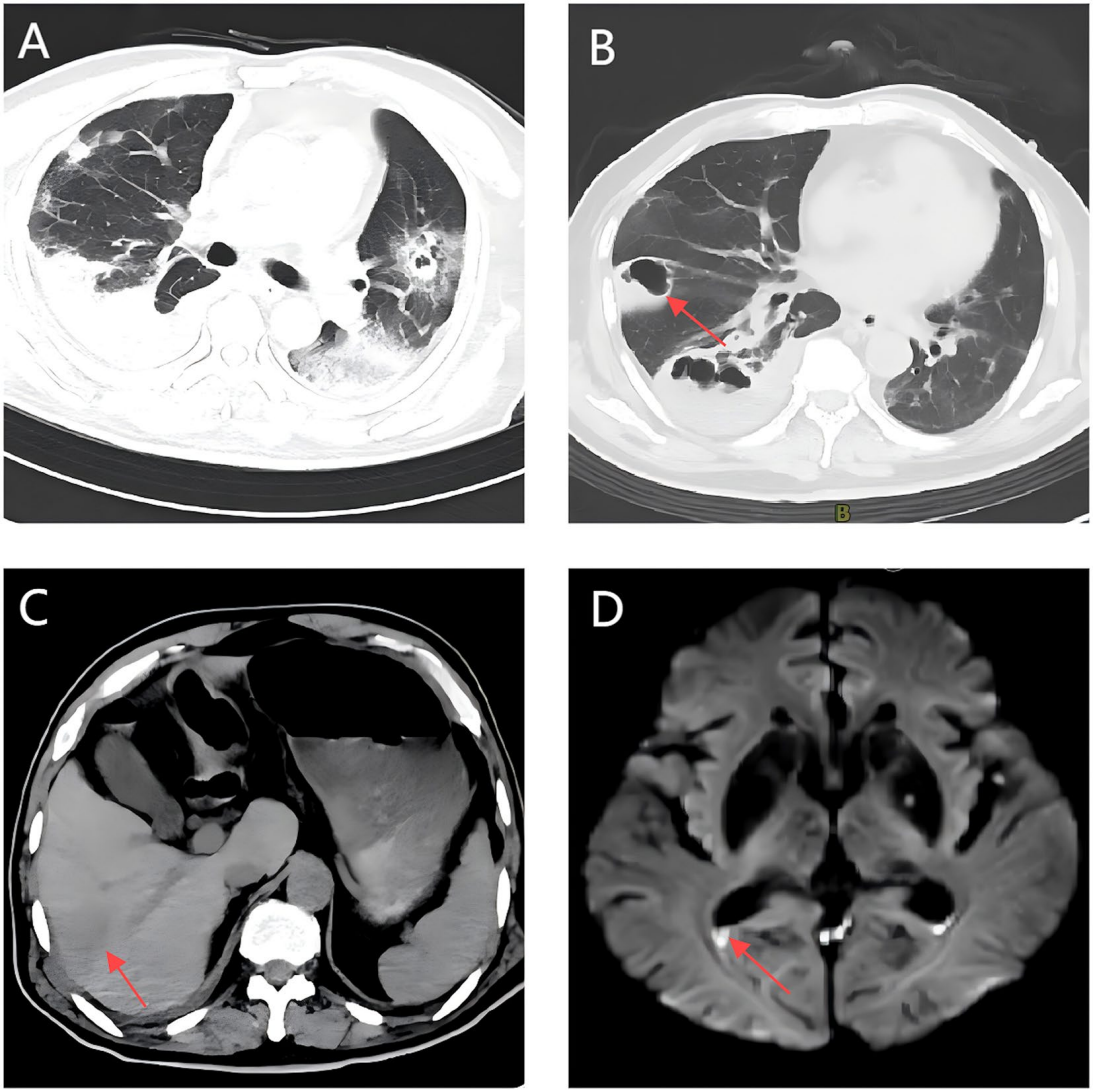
Follow-up multimodal imaging findings during treatment: Chest CT, Abdominal CT, and Brain MRI. (A) A Chest CT scan shows bilateral cavitary pneumonia with right pleural effusion. (B) Multiple thin-walled pulmonary abscesses with rim enhancement (arrow). (C) Hepatic abscess (segment 6) with hepatic vein thrombosis (arrow). (D) Bilateral ring-enhancing cerebral lesions with DWI hyperintensity in ventricular posterior horns (arrow).
